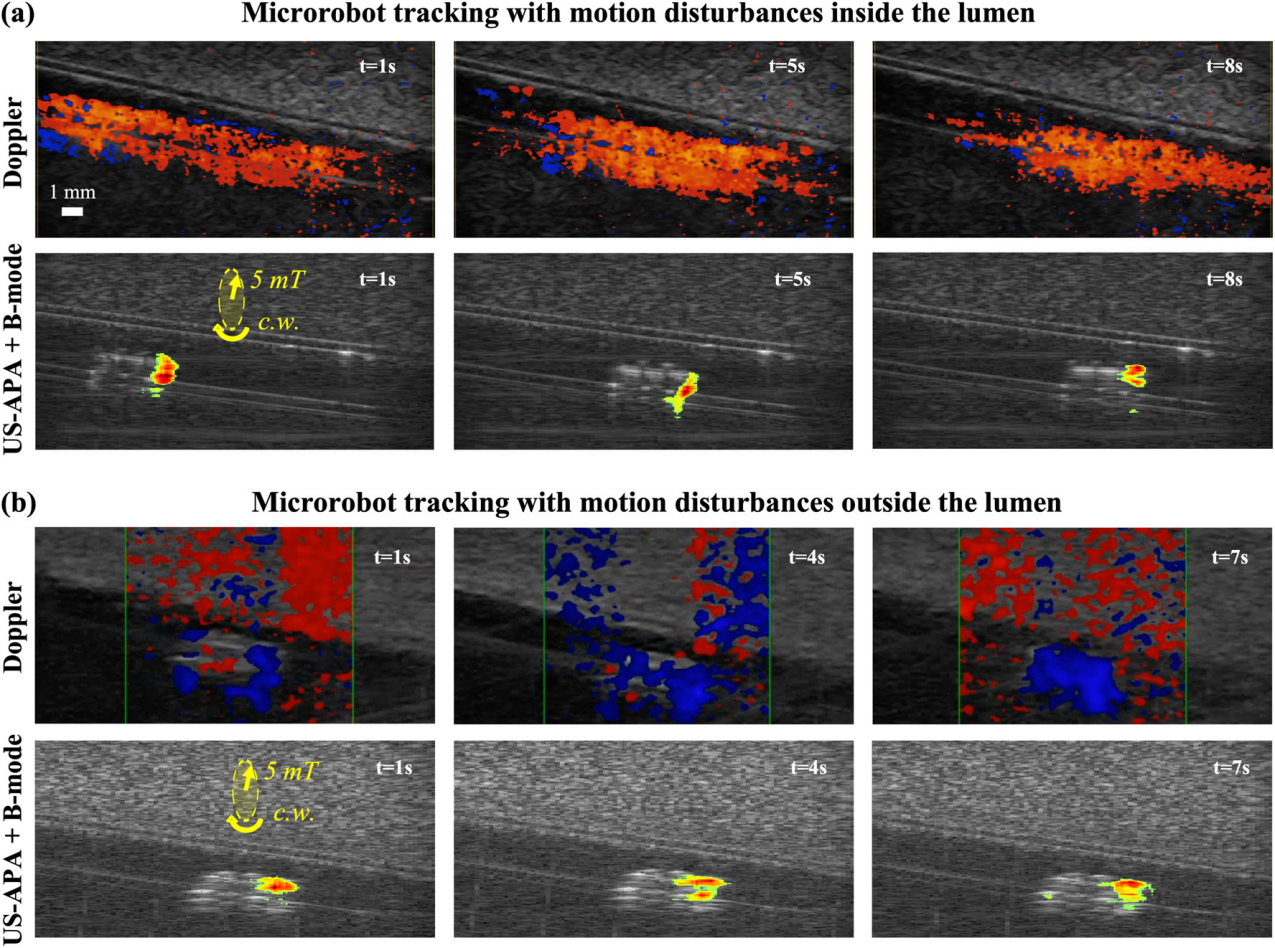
Dynamic tracking experiments in the presence of motion disturbances. Yellow arrows represent the field intensity and rotation direction (c.w. stands for clockwise). (a) Images acquired at different time instants with both color Doppler and US-APA + B-mode during MR navigation against fluid flow in the lumen. (b) Images acquired at different time instants with both color Doppler and US-APA + B-mode during MR locomotion when surrounding tissue motion occurred due to repositioning of the US transducer.
